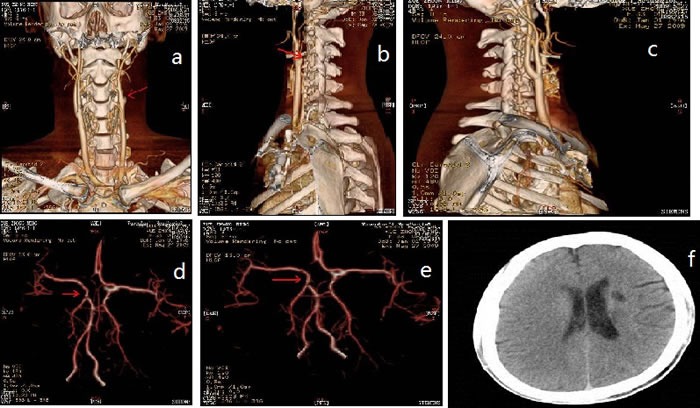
CT-angiography and brain magnetic resonance imaging (MRI) Red arrows: multifocal infarction
